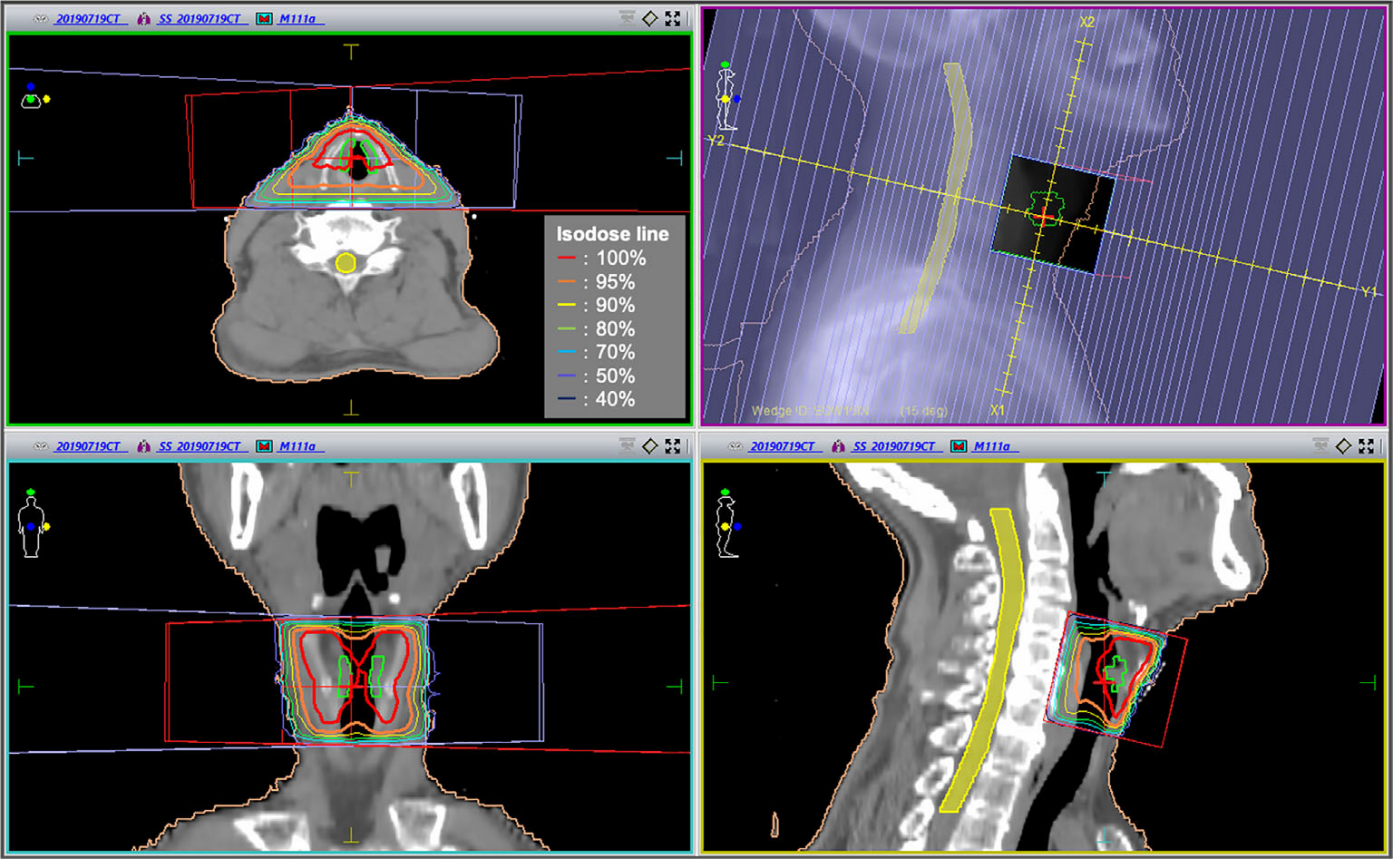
Planning CT image of RT. The RT technique was a three‐dimensional conventional technique using a dynamic wedge. The radiation field size was 5.5 × 6 cm with a conventional rectangle field (no multi‐leaf collimator). CT, computed tomography; RT, radiotherapy
